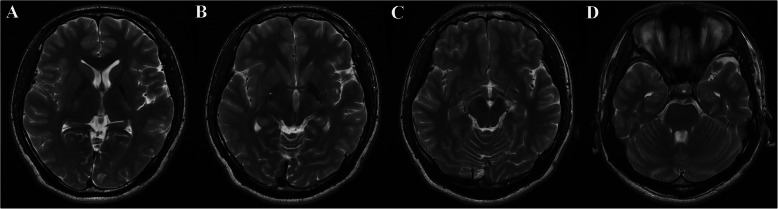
T2-weighted images of the patient. Subcortical and brainstem structures show atrophic changes in the left basal ganglia (a), thalamus (b), midbrain (c), and pons (d). Furthermore, dense hypointensity was seen in the left globus pallidus (a, b), with light hypointensity in the left substantia nigra (c)
